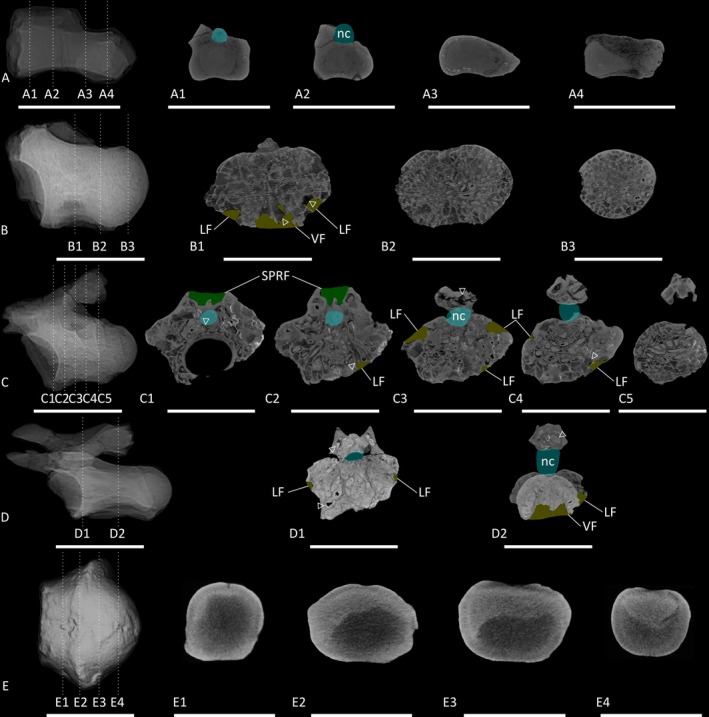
CT scan of saltasaurine sauropod caudal vertebrae. (A–D) CT scan left lateral view, (E) SEM scan dorsal view; (A) *Neuquensaurus australis* middle caudal centrum MCS‐Pv 5/8, (B) *Rocasaurus muniozi* anterior caudal vertebra MPCA‐Pv 47, (C) *Rocasaurus muniozi* middle caudal vertebra MPCA‐Pv 57, (D) *Rocasaurus muniozi* middle caudal vertebra MPCA‐Pv 58, (E) *Yamanasaurus lojaensis* middle caudal vertebra YM‐INPC‐014; (A1–A4, B1–B3, C1–C5, D1, D2, E1–E4) CT scan slices in transverse cross‐section. LF, lateral fossa; nc, neural canal; SPRF, spinoprezygapophyseal fossa; VF, ventral fossa. Key: Blue highlight, neural canal; green highlight, neural spine fossa; yellow highlight, centrum fossa; white arrow, pneumatic bone texture. Scale bars = 100 mm.
